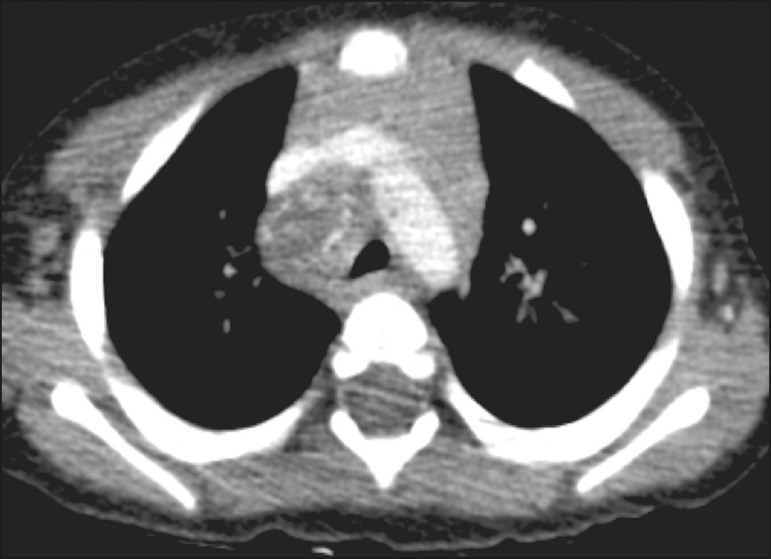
Contrast-enhanced CT, with soft-tissue window settings, of na
